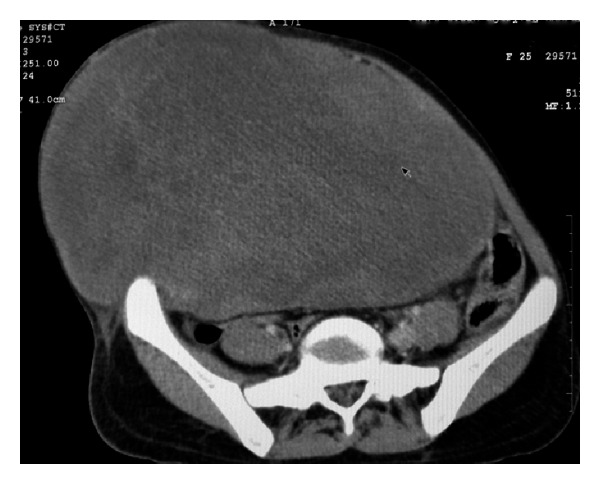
CT scan showing mass arising from anterior abdominal musculature.
